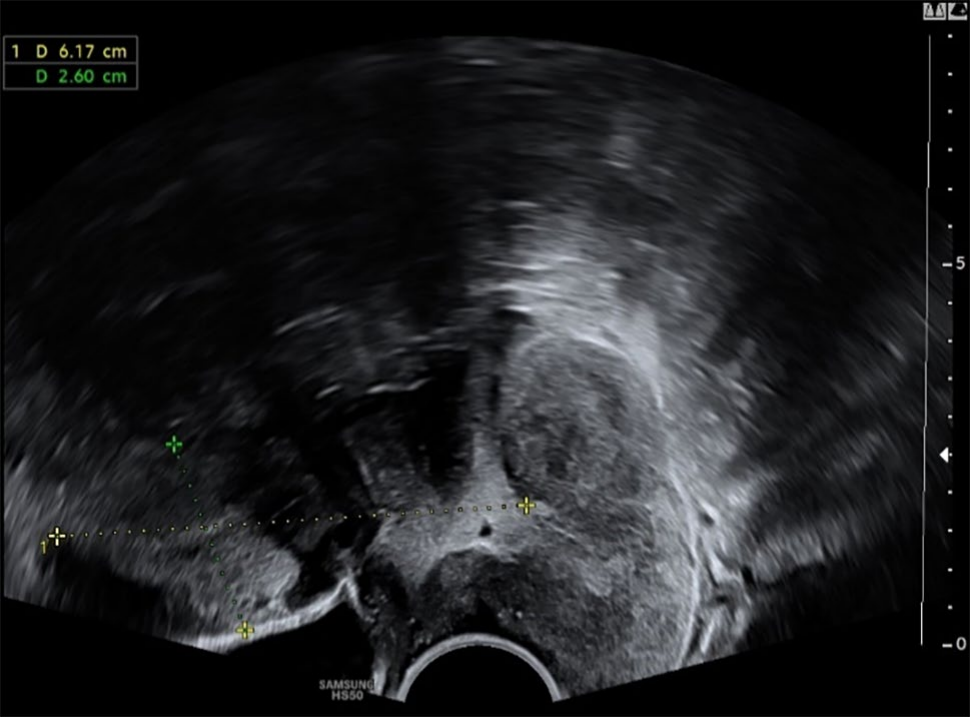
Transvaginal sonogram of the uterus and tumor of the cervix
